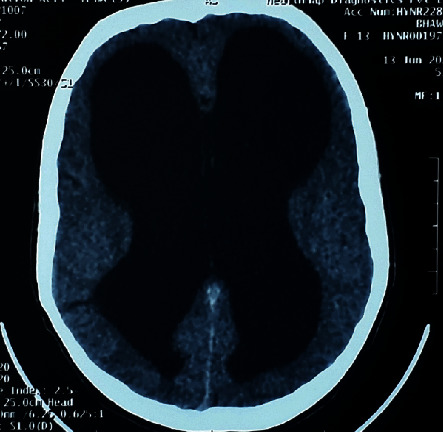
Computed tomography of the brain, axial section showing dilated ventricles suggestive of hydrocephalus.
